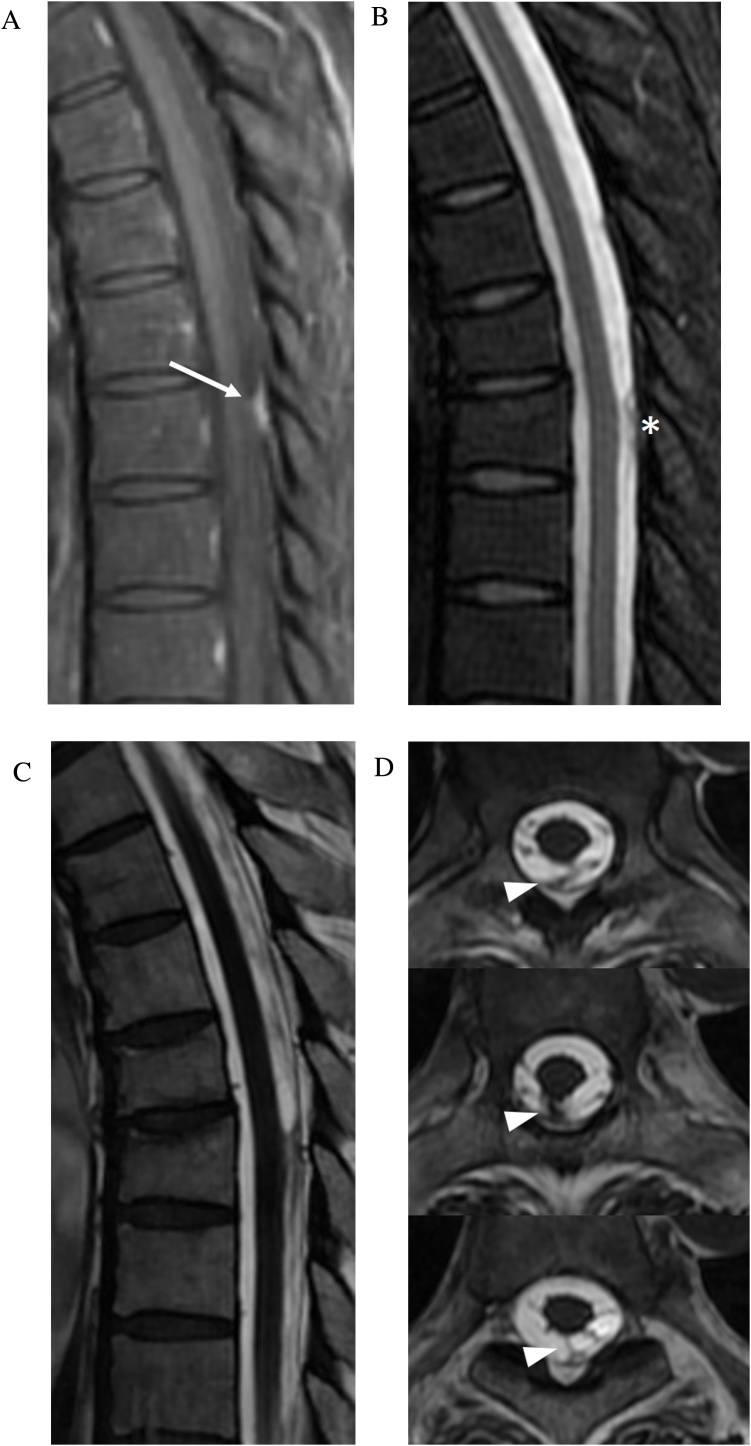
Arachnoid scar. 46-year-old man status post radiation therapy for nasopharyngeal carcinoma, complicated by intracranial abscesses. Sagittal contrast enhanced fat saturated T1-weighted FSE image (slice thickness, 3 mm) of the thoracic spine (a) demonstrates a focus of enhancement along the dorsal thecal sac (arrow), which could be interpreted as an metastasis. Sagittal T2-weighted fat saturated FSE image (slice thickness, 3 mm) (b) shows an irregular hypointense nodule (asterisk). Sagittal and axial FIESTA-C MRI images (c–f) resolves the lesion as irregular linear bands of low signal within the dorsal subarachnoid space (arrowheads) consistent with arachnoid scar.
